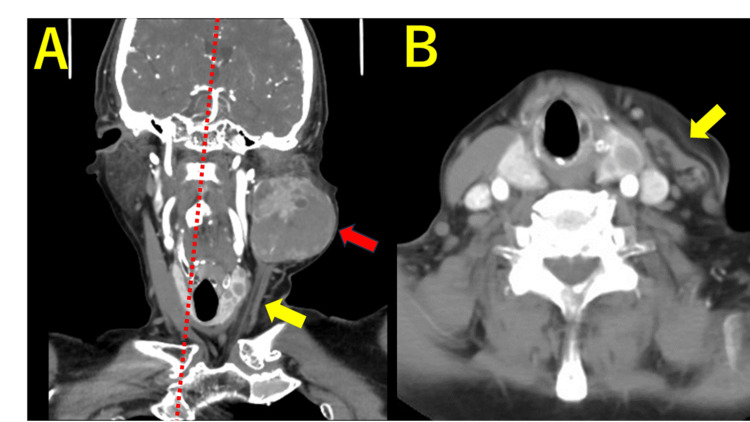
Preoperative CT images (A) Coronal section. A left parotid tumor is observed (red arrow). Atrophy of the left SCMM (yellow arrow) and oblique neck (red dotted line) are noted. (B) Axial section. Atrophy of the left SCMM (yellow arrow) is noted. SCMM, sternocleidomastoid muscle
